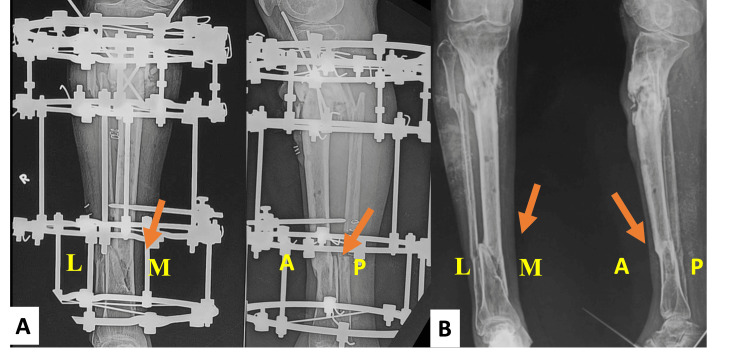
A) Radiograph of the AP and lateral view taken before frame removal of the segmental tibia fracture with upper metadiaphyseal fracture and lower diaphyseal fracture of the tibia treated by Ilizarov frame. Region of interest: Marked in orange arrow - lower third tibia diaphyseal fracture. m-RUST score of medial = 3; lateral = 3; anterior = 4, and posterior = 4. The total m-RUST score assessed is 14. B) AP and lateral view radiographs of the leg showing healed fracture of lower third tibia diaphyseal fracture (marked with an orange arrow) for reference calculation of the m-RUST score. AP - anterio-posterior; M - medial; L - lateral; A - anterior; P - posterior
